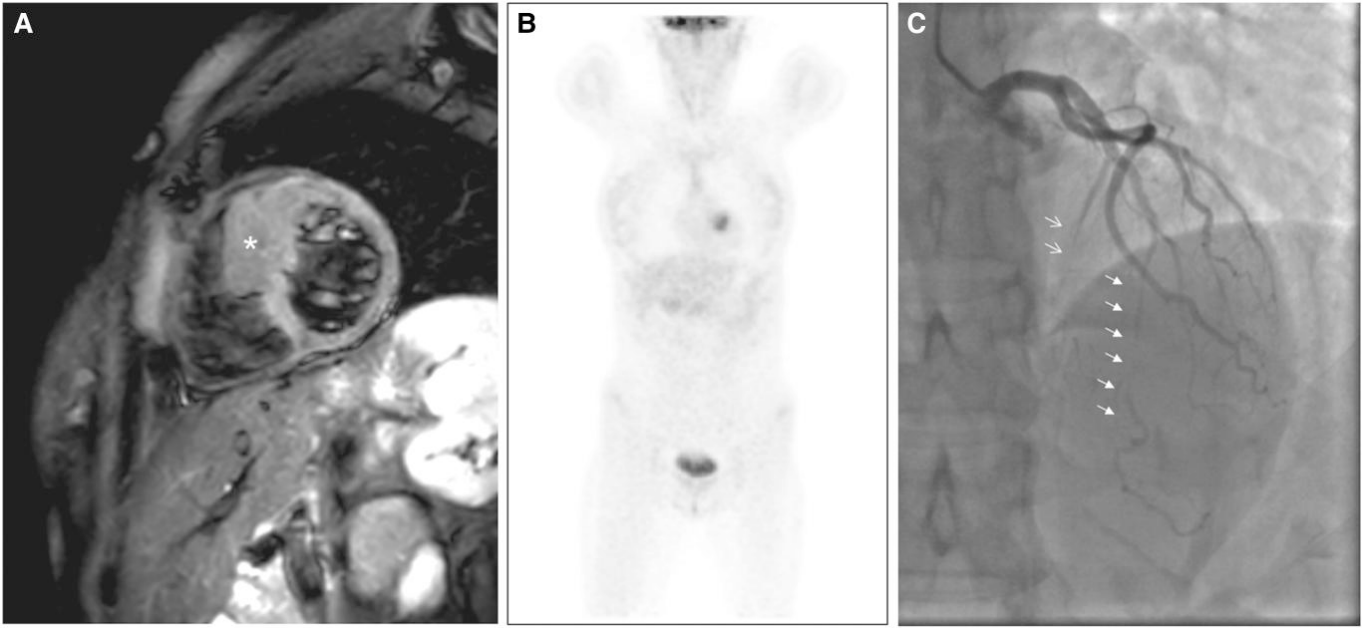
(*A*) T2* MRI image showing the mass (*) as a bulging of the interventricular septum with areas of inhomogeneous intensity. (*B*) ^18^F-FDG uptake of the mass, localized in the interventricular septum. (*C*) Coronary angiogram: ‘squeezing' of the first septal branch against the HMCM during systole (slant open arrows); ‘milking’ of the LAD due to a myocardial bridging (closed arrows) is also shown. HMCM: hamartoma of mature cardiac myocytes; LAD: left anterior descending artery.
